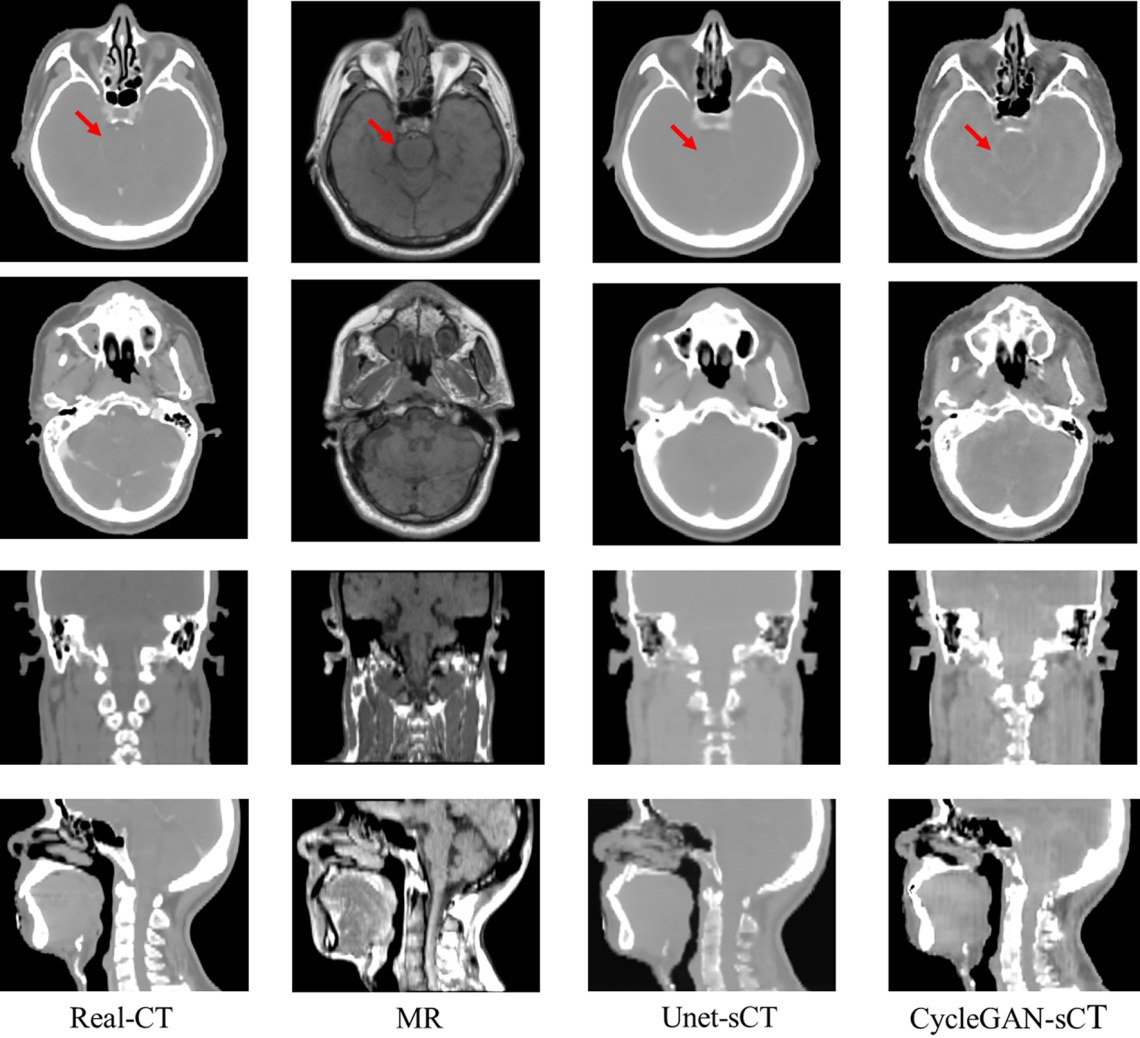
Real CT, MR, and synthetic images in axial, sagittal, and coronal positions. The window width and window level are 1000 and -60HU, respectively. The red arrow points out the location of the brain stem
